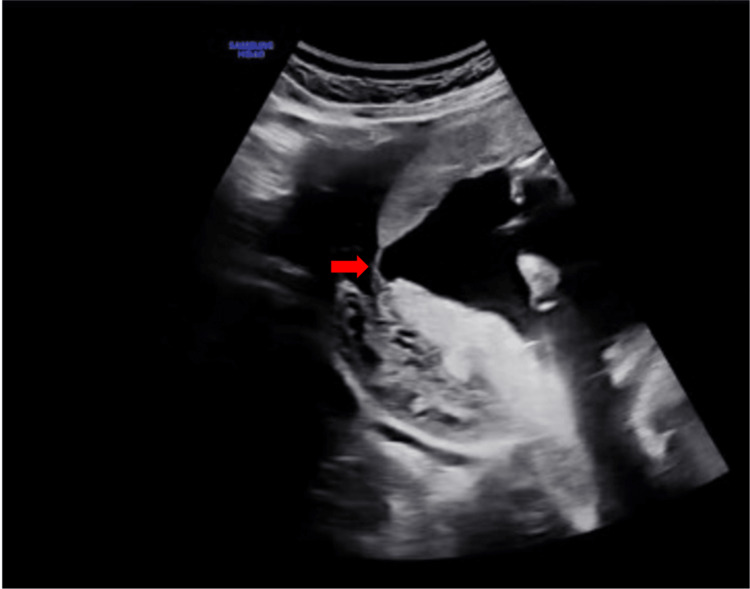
Ultrasound image showing the incomplete uterine rupture (red arrow showing that the myometrium is disrupted)
